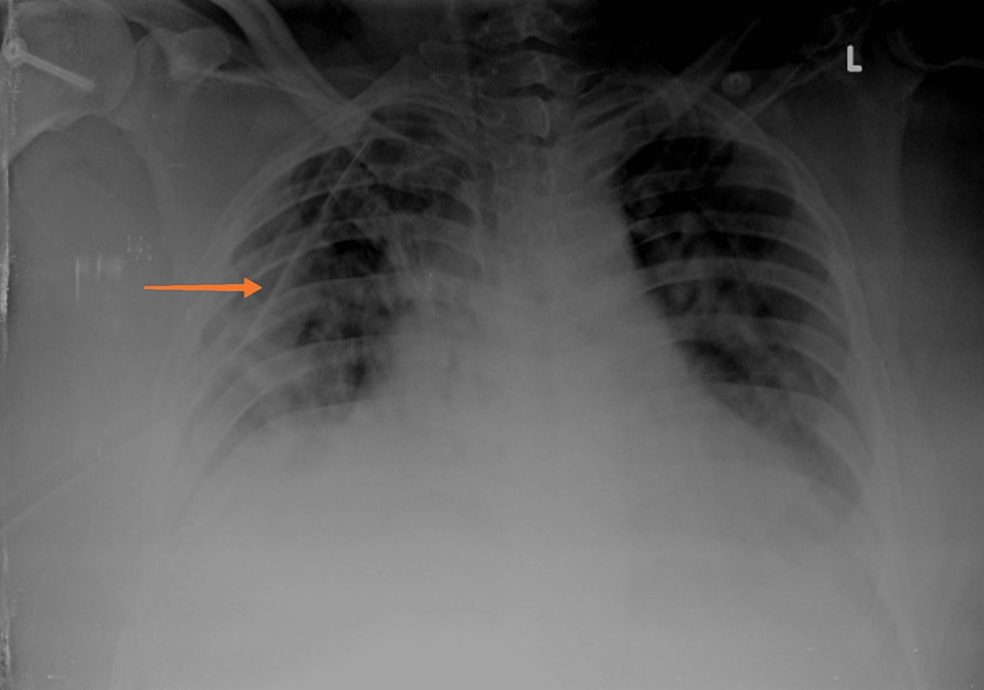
Chest X-ray: pneumothorax with ICD in situ in the right pleural space. ICD, intercostal drain
